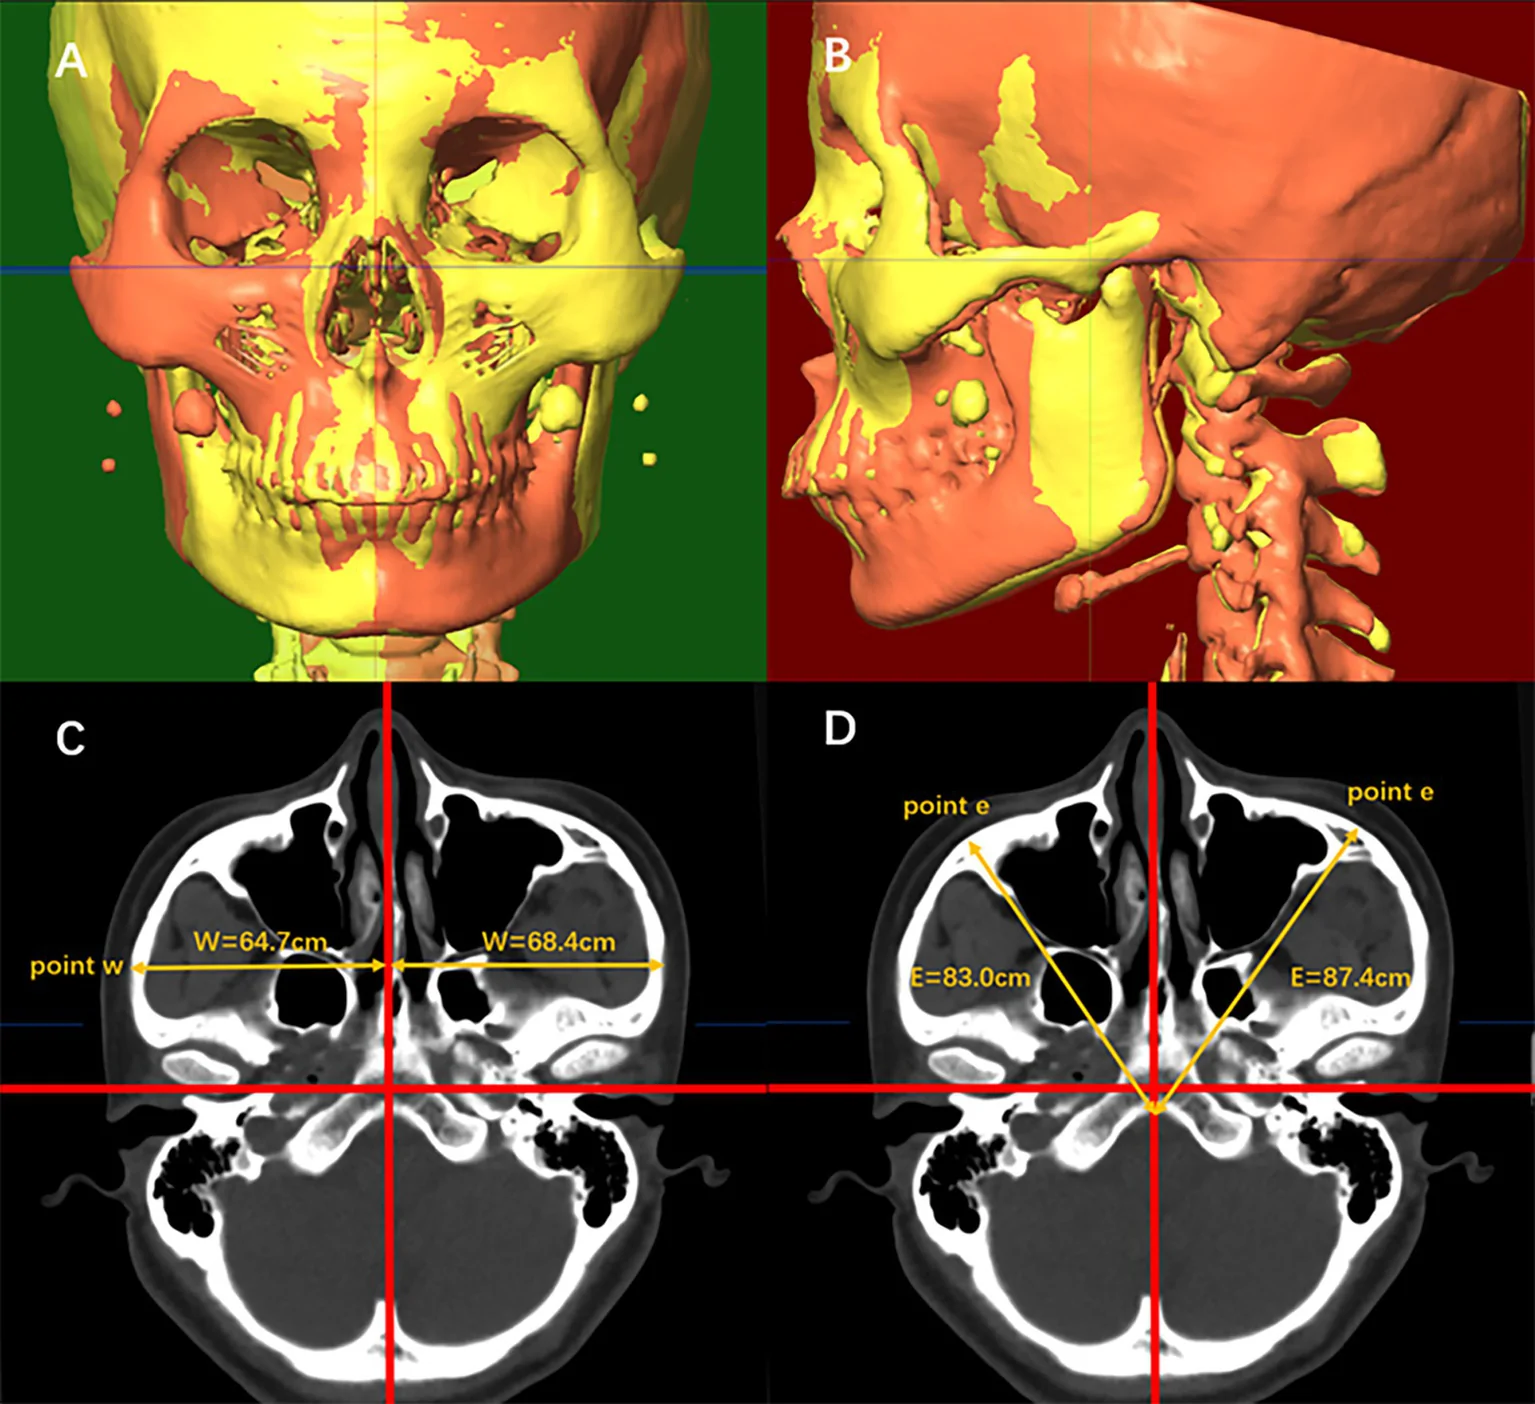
CT-based morphometric assessment of zygomatic asymmetry in a representative Type III lesion. (A,B) Three-dimensional CT reconstructions show mirrored craniofacial models and the midsagittal reference plane. (C) Zygomatic width (*W*) was measured from the midsagittal plane, and the bilateral width difference was recorded as *DW*. (D) Zygomatic eminence (*E*) was measured relative to the coronal reference plane, and the bilateral eminence difference was recorded as *DE*. *DE* exceeded the 4-mm threshold for clinically significant asymmetry.
